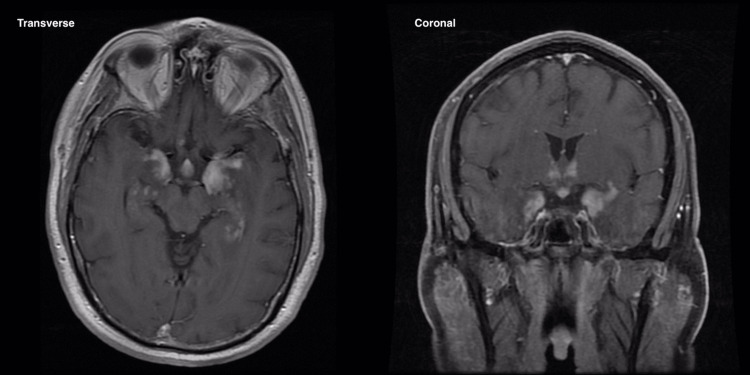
Transverse (left) and coronal T1-weighted MRI (right) Showing further contrast enhancement of the lesions MRI: Magnetic resonance imaging
